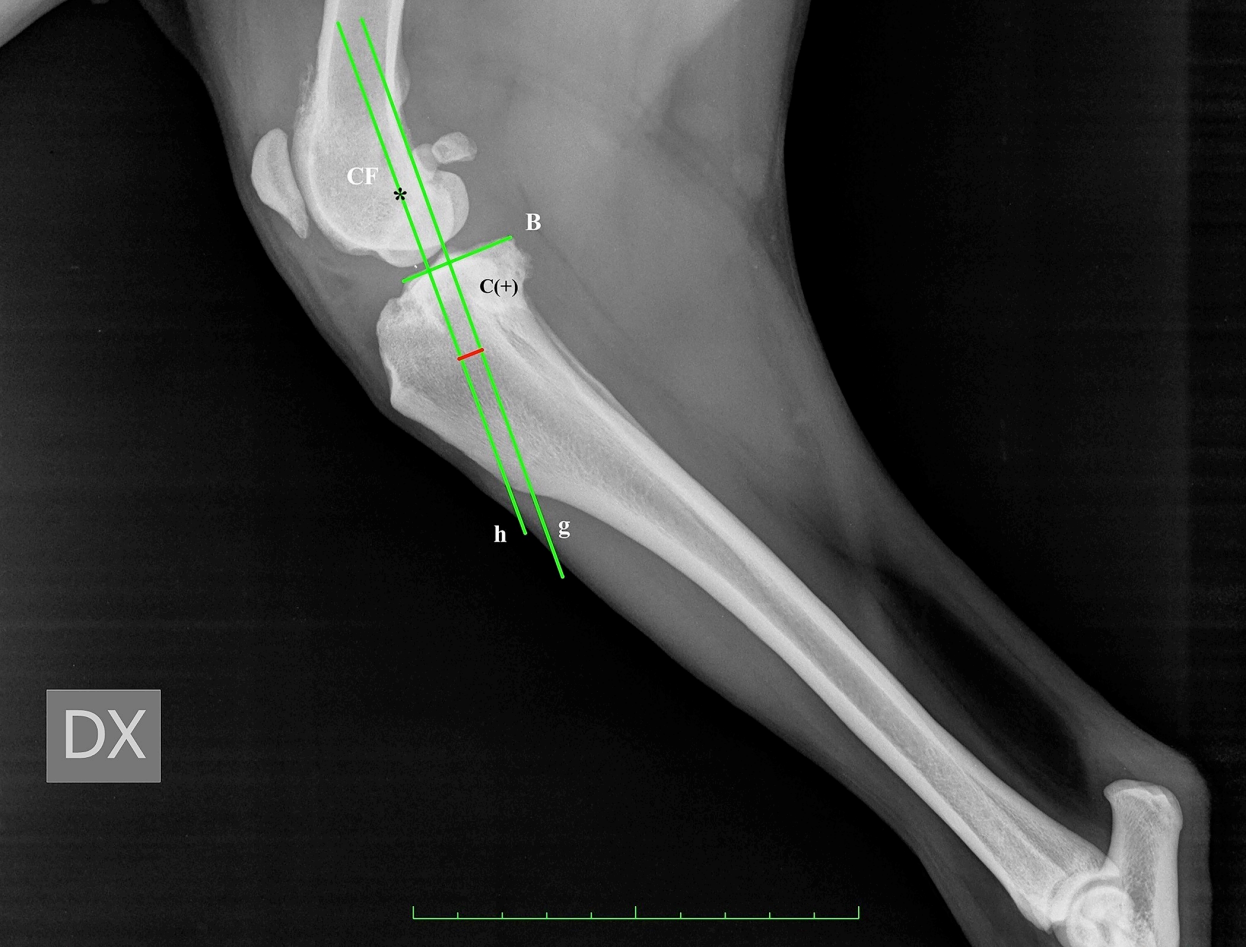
Radiographic landmarks of BM. Center of the femoral condyle (CF), length of the tibial plateau (B), g: line passing through intercondylar eminence, perpendicular to the tibial plateau; h: line passing through CF, perpendicular to the tibial plateau, distance C detect the subluxation value.
